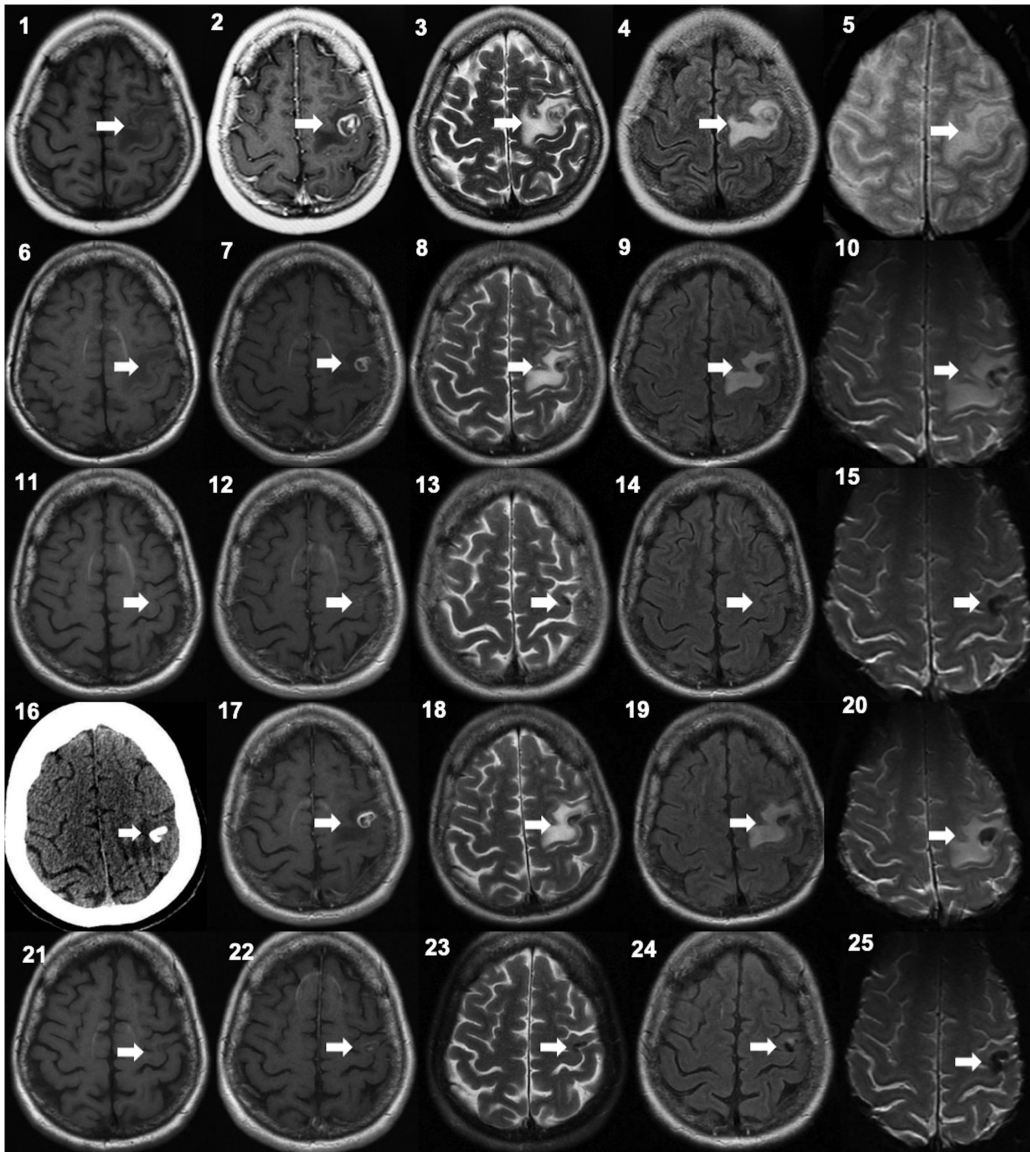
Neuroimaging showing the findings of the initial diagnosis of cerebral toxoplasmosis and the first and third subsequent episodes of perilesional edema and enhancement around a calcification secondary to this opportunistic disease. In the first row: initial MRI (10/2010) with the lesion located in the precentral gyrus. (1) T1-weighted image, before contrast administration and (2) post-gadolinium image showing ring enhancement with an eccentric nodule in this lesion; in (3) and (4), T2 and FLAIR images, respectively, showing associated edema in the surrounding white matter. In (5), T2* image (GRE), with no signs of calcification or hemorrhage in this lesion; In the second row: MRI of the first episode of perilesional edema associated with calcification (11/2015). T1-weighted image showing the previous lesion with a smaller size, (6) before and (7) after gadolinium; in (8) and (9), T2 and FLAIR images, respectively, showing associated edema in the surrounding white matter. In (10), T2* image (GRE), some low signal areas can be seen, which may represent early calcium deposits; In the third row: following MRI (03/2016) showing resolution of the first episode of perilesional edema associated with calcification. T1-weighted images, (11) before and (12) after gadolinium, showing significant regression of the lesion enhancement and T2 (13) and FLAIR (14) images without edema. In (15), T2* image (GRE), better definition of the pronounced low signal areas, suggesting intensification of the calcium deposits in the lesion site; In the fourth row: CT and MRI (12/2021) of the last episode of perilesional edema associated with calcification. (16) CT showing calcification in lesion site with surrounding edema and (17) MRI T1-weighted post-gadolinium image with lesion enhancement, as well as increased perilesional edema in T2 (18) and FLAIR (19) images. In (20), T2* image (GRE), increasing of the pronounced low signal areas, compatible with the calcification showed in the concomitant CT scan (16); In the fifth row: subsequent MRI (04/2022) of the third episode of perilesional edema associated with calcification. T1-weighted images, (21) before and (22) after gadolinium, showing resolution of the inflammatory activity with minimal enhancement and the disappearance of the edema in the T2 (23) and FLAIR (24) images. In (25), T2* image (GRE) showing persistence of pronounced low signal areas, compatible with calcification (16).
